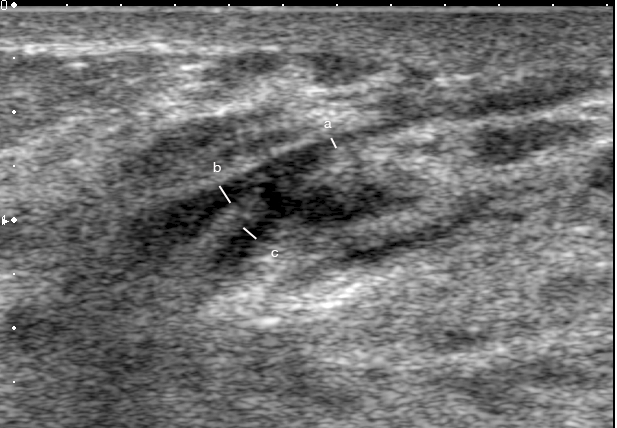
Ultrasound image of the main milk duct in a lactating woman. The milk ducts are displayed on ultrasound as hypoechoic (black) branching structures. The measured main milk duct is barely imperceptible with a diameter of 0.4 mm (a). The merging milk ducts measured 0.8 mm (b) and 0.75 mm (c).
